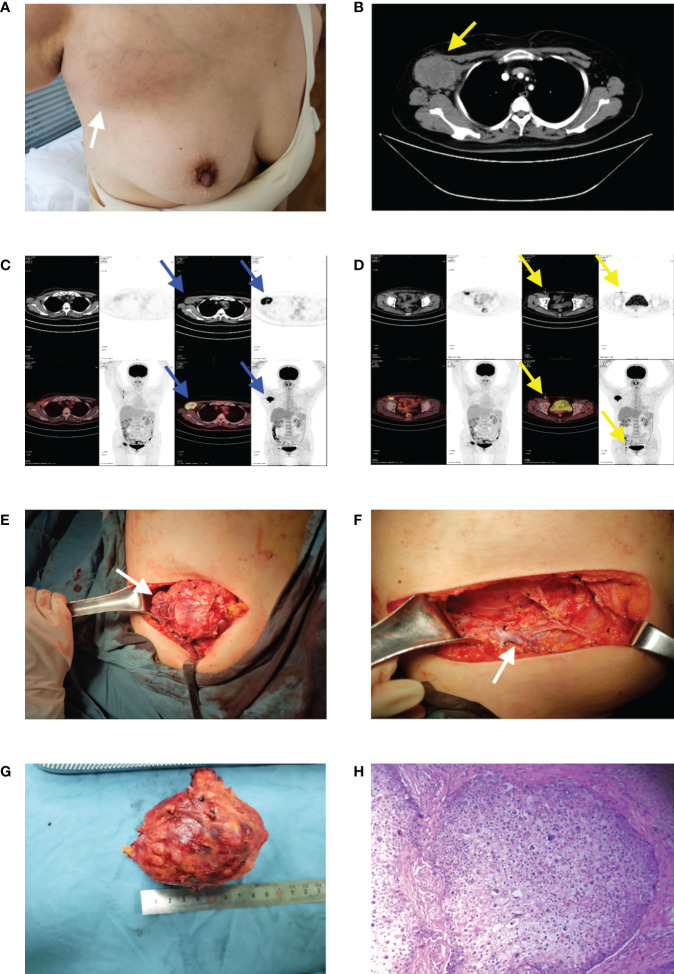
(A) A mass in the right axilla. (B) Preoperative enhanced CT revealed a soft tissue density mass (yellow arrow) in the right axilla with a size of 6.9 × 5.2 cm. (C, D) PET-CT showed the mass as axillary lymph node metastasis (blue arrows) and another metastasis located at the ipsilateral inguinal lymph node (yellow arrows) which were characterized by shapes of nuclide accumulations in PET-CT image. (E) An intraoperative view from above, showing the tumoral invasion of the pectoralis major and minor muscles (white arrow). (F) An intraoperative view from above, showing the axillary vein (white arrow) after ALND. (G) The tumor was 7 cm in diameter. (H) The HE ×100 showed the tumor was characterized by a proliferation of tumoral lobules composed of large, atypical cells with clear cytoplasm.
